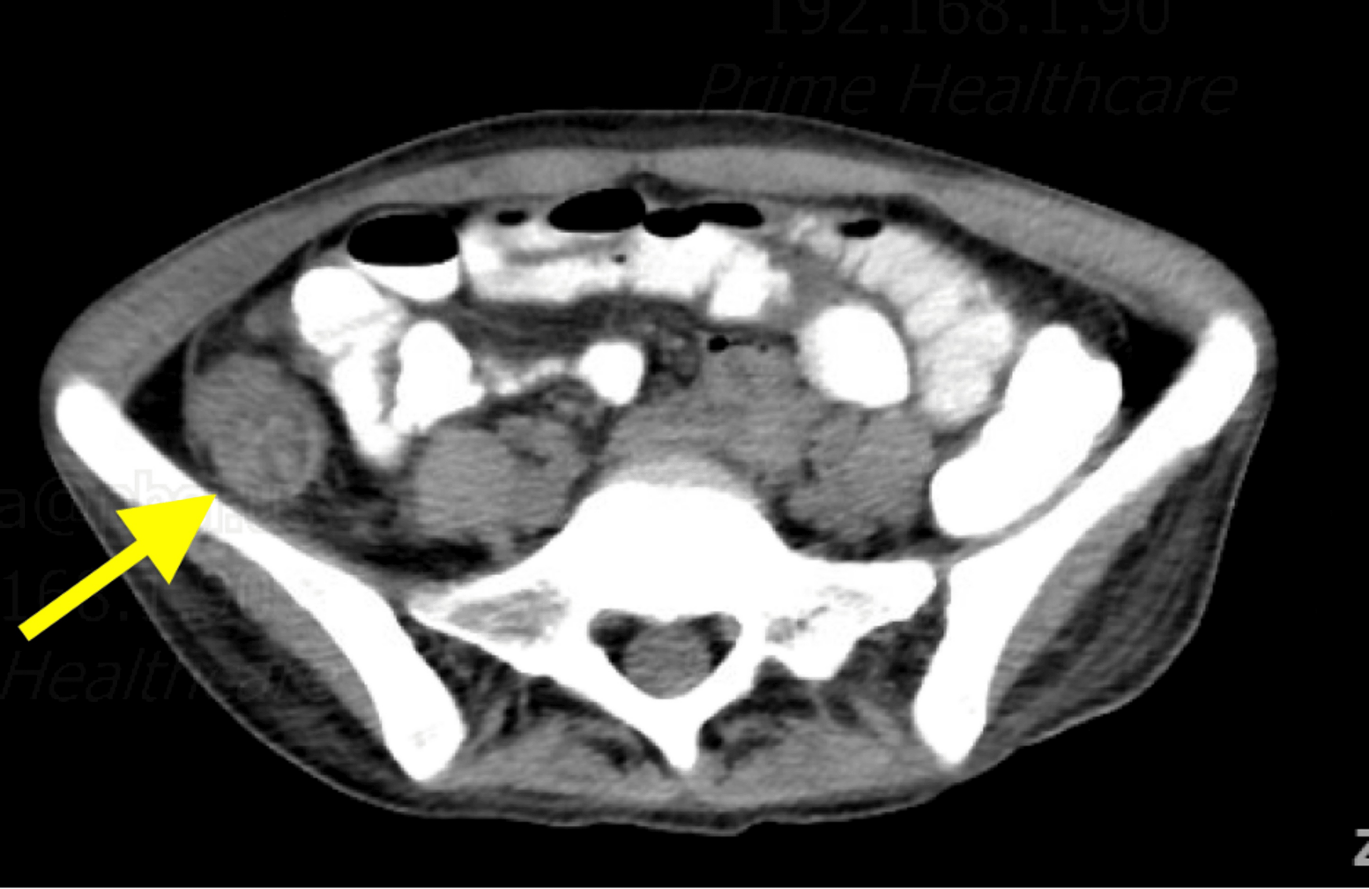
CT of the abdomen and pelvis revealing wall thickening of the ascending colon (yellow arrow).
